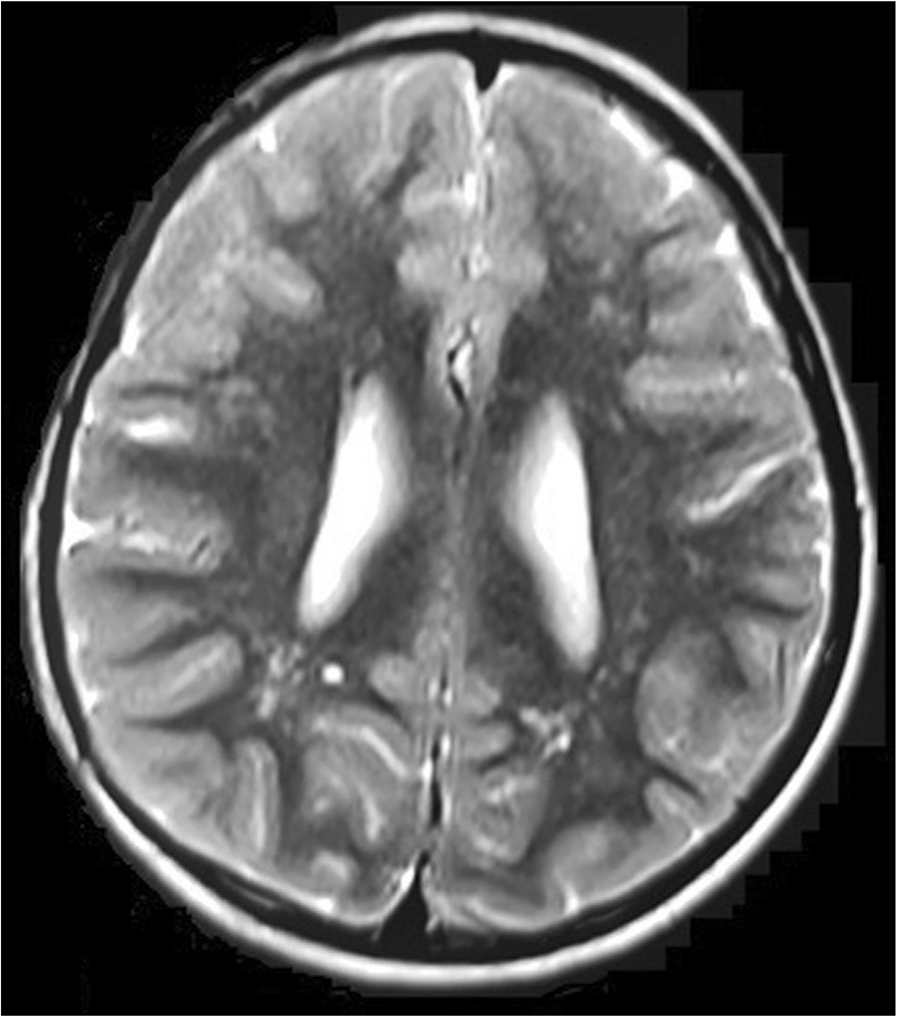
MRI picture depicting bilateral frontal and parietal lobe white matter changes
